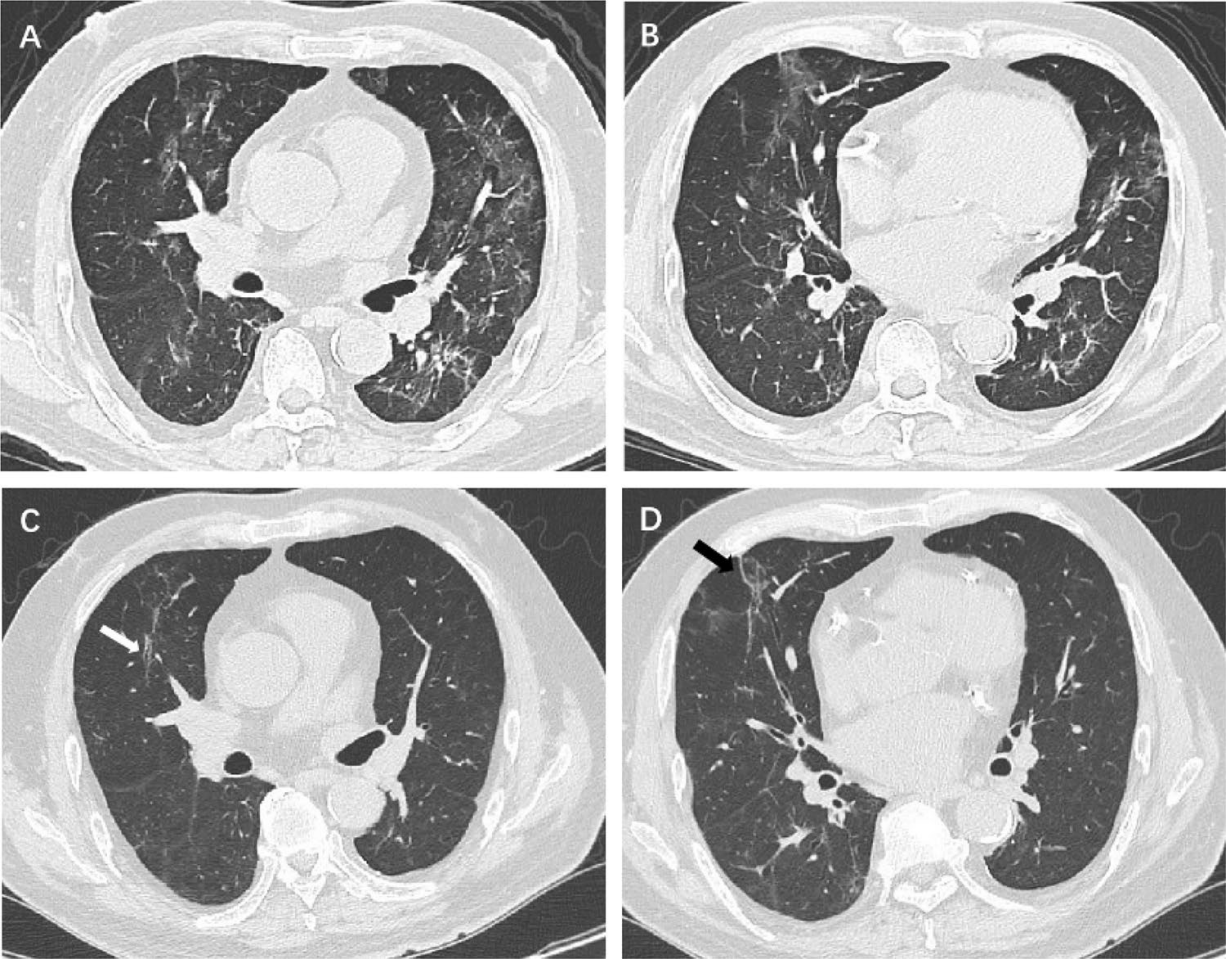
Serial chest CT scans in a 60-year-old man with coronavirus disease 2019 pneumonia. (A, B) Initial CT scans obtained on day 8 after the onset of symptoms showed multiple ground-glass opacities and interstitial thickening bilaterally. (C, D) CT scans obtained on day 180 showed traction bronchiectasis (white arrow) and interlobar pleural traction (black arrow) in the upper lobe of right lung.
